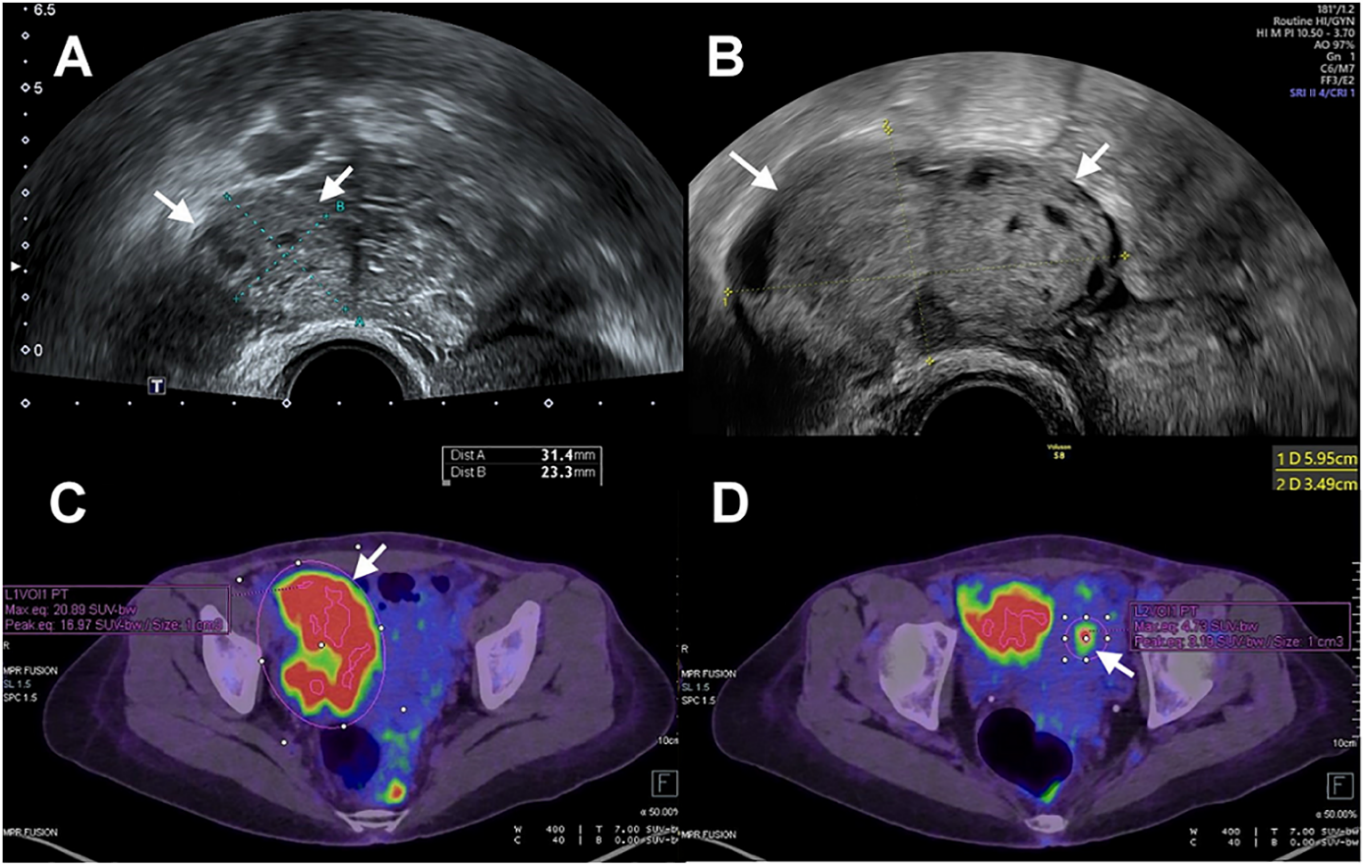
(A) Transvaginal ultrasonography showed a right ovary (arrow) measuring 3 cm in size. (B) The size of the right ovary (arrow) increased from 3cm to 6 cm with a three-and-a-half-month interval between examinations. (C) PET scan showed increased FDG accumulation of right ovary (arrow). (D) PET scan showed increased FDG accumulation of uterine cavity (arrow).
